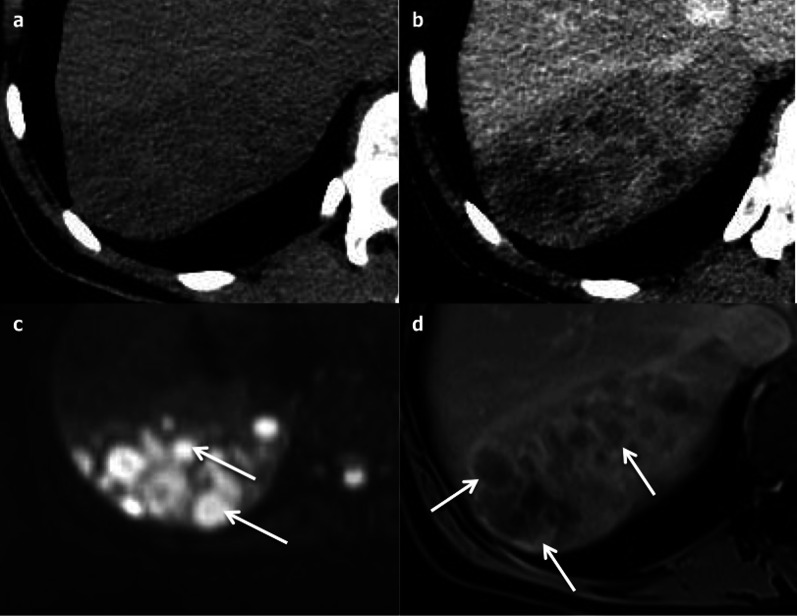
Invasive mucinous cystic neoplasm of the liver in a 50-year-old female. a Axial non-enhanced computed tomography shows a hypoattenuating lesion with a thick heterogeneous enhancing wall on portal venous phase (b), better seen on T1-weighted magnetic resonance imaging on portal venous phase (d) (arrows). Note the presence of cystic portions on axial T2-weighted sequence (c), in very intense hypersignal T2 (arrows)
